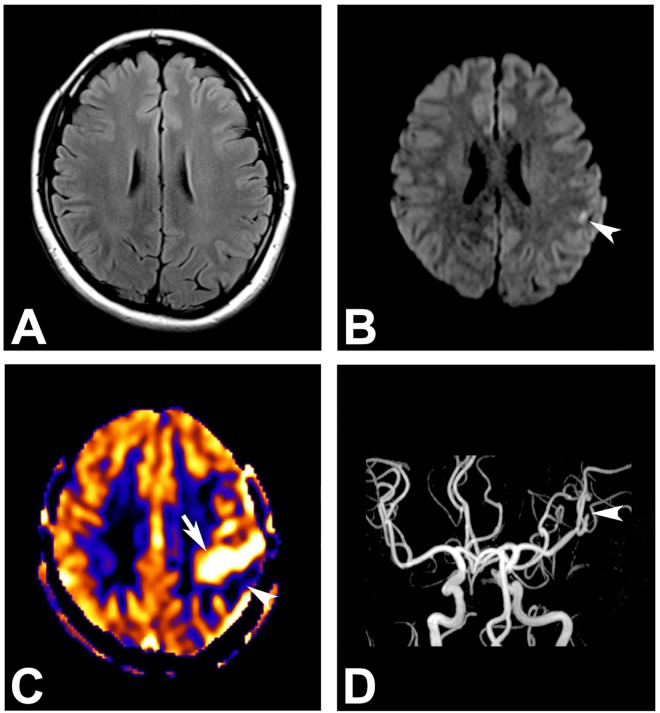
A 33-year-old woman who visited the emergency department for a nonconvulsive seizure. (A) Brain parenchyma appears normal on the T2 FLAIR image. (B) A tiny dot-like diffusion restriction is noted at the left parietal cortex (arrowhead). (C) Arterial spin labeling perfusion-weighted MR image depicts hyperperfusion at the left middle cerebral artery territory (arrow). Note the combined hypoperfusion portion adjacent to the hyperperfused area (arrowhead). (D) MR angiography reveals occlusion at the left M2 segment (arrowhead).
